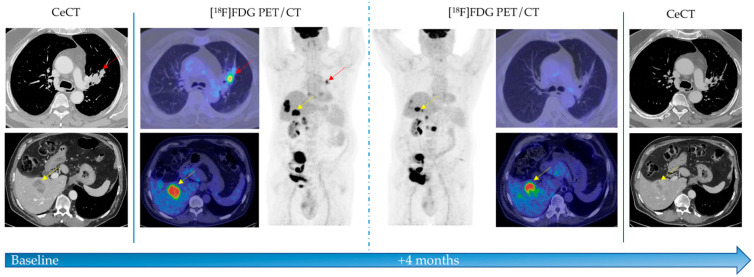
A 60-year old man affected by non-small cell lung cancer underwent [^18^F]FDG PET/CT before and after immunotherapy. PET/CT showed high [^18^F]FDG uptake into primary lesion in the left lung (red arrows) and in the liver (yellow arrows), as secondary disease localizations. After four pembrolizumab cycles, patient underwent morphological and functional revaluation. [^18^F]FDG PET/CT showed no more uptake in primary tumor and a reduction of metabolic activity in liver lesions. CeCT evaluation confirmed partial response (PR) to immunotherapy. CeCT: contrast enhanced computed tomography; [^18^F]FDG PET: Fluorine-18 fluorodeoxyglucose positron emission tomography.
